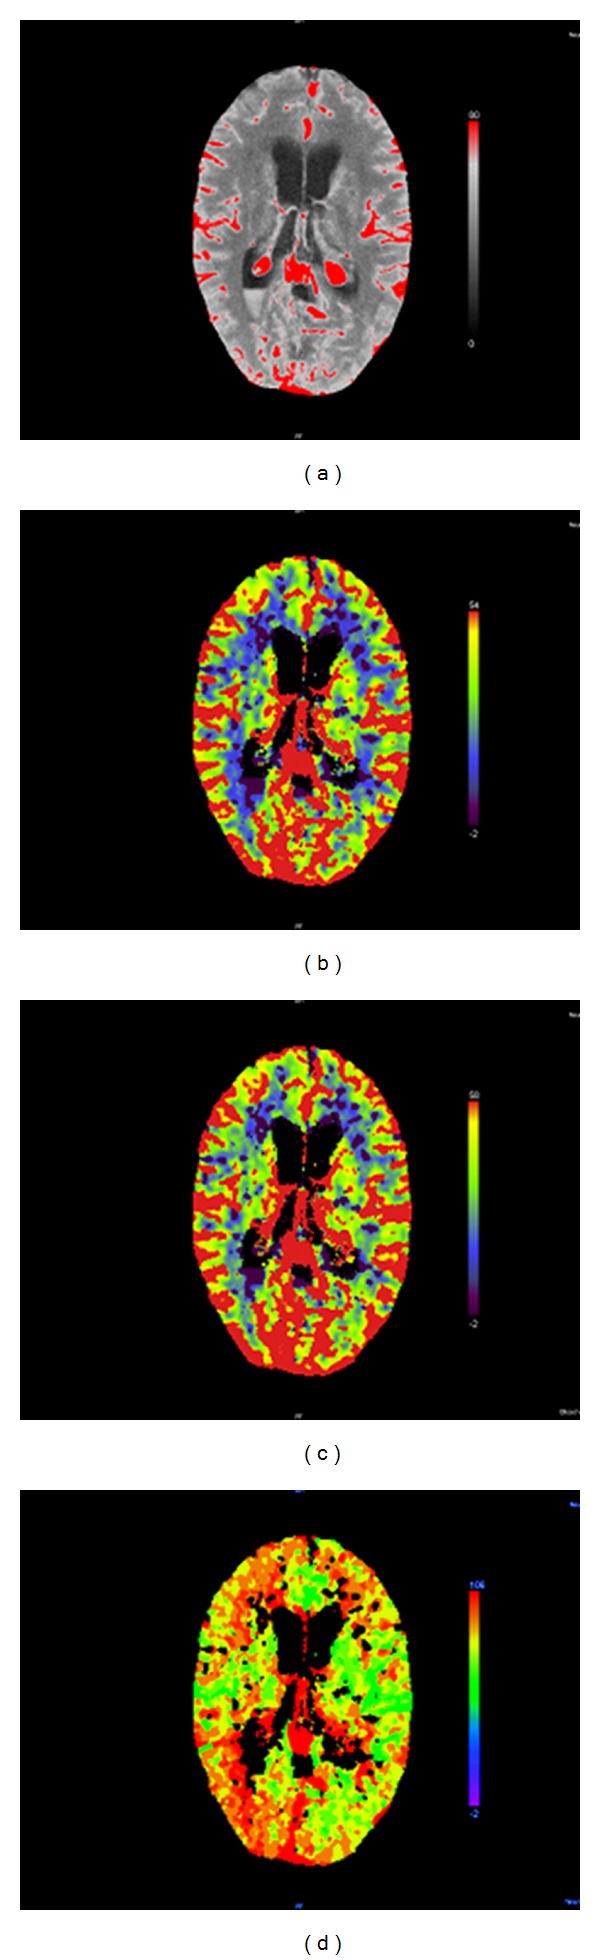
Perfusion-CT of a 40-year-old female patient with subarachnoid hemorrhage Hunt/Hess 3 caused by rupture of a right posterior communicating artery aneurysm. (a) Basis scan, (b) CBF map, (c) Blood Volume map, and (d) time to peak map. While the CBF map and Blood Volume map do not show signs of a perfusion deficit, the TTP map depicts an asymmetry in the posterior MCA-territory indicating spasm of the right terminal carotid artery and MCA.
